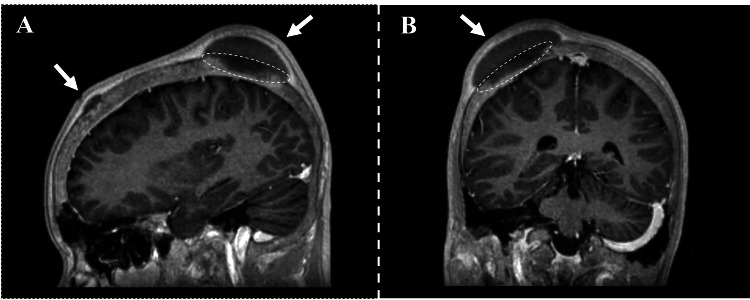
Sagittal (A) and coronal (B) MRI with contrast. Hypointense signals of the right parietal and anterior frontal calvarium suggest bony infarction. The bone infarctions are indicated with circles, and the adjacent hematomas are indicated with arrows.
